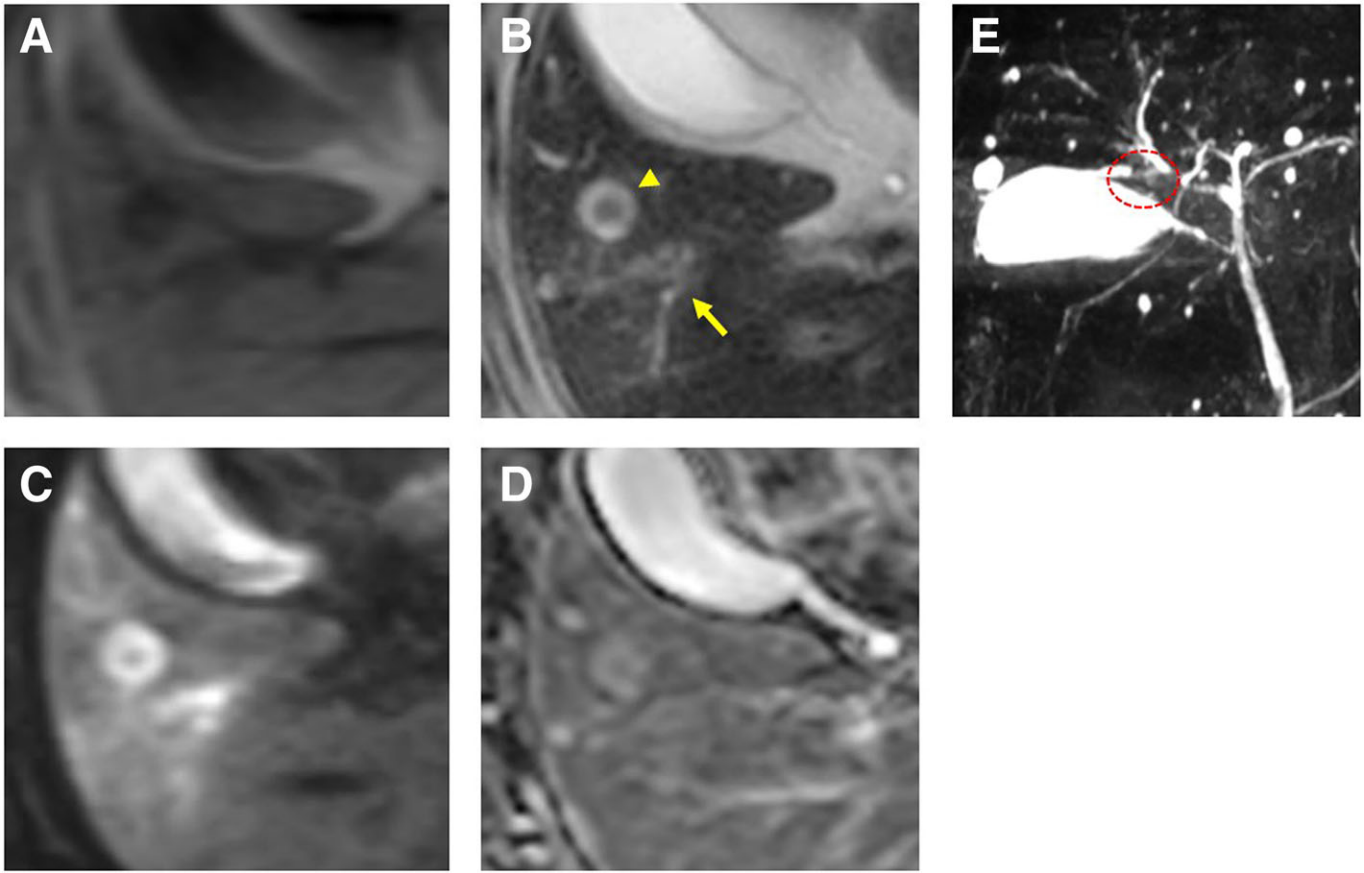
a-e:a: MRI T1WI: There was hypo-intensity around the dilated bile duct. b: MRI T2WI: Periductal high-intensity was observed around the dilated bile ducts (arrow) and the high-intensity area included a low-intensity area in S5 (arrowhead). c: MRI DWI: The dilated bile ducts showed periductal high intensity. d: MRI ADC map: Slight high-intensity was observed around the bile ducts. e: MRCP showed stenosis of the anterior-inferior branch of the bile duct (red circle)
